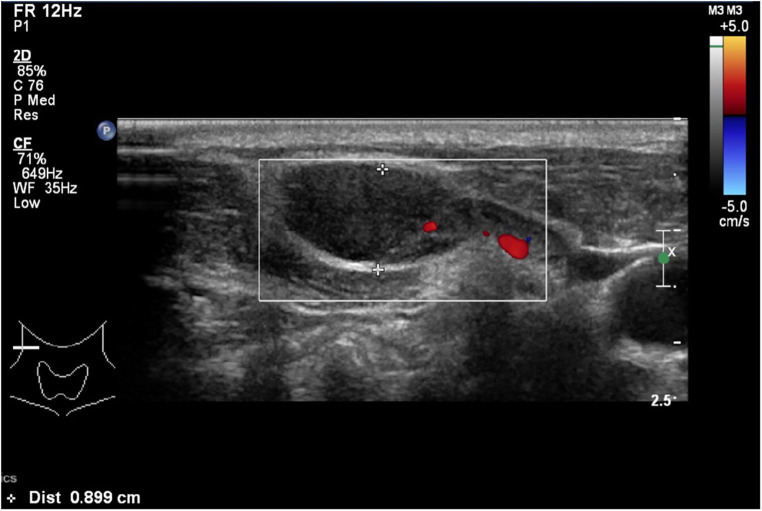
A neck ultrasound showing an enlarged lymph node which measures 1.9 cm in transverse diameter, 0.8 cm in anteroposterior diameter and 2.2 cm in crania-caudal diameter in the right upper posterior triangle region.
